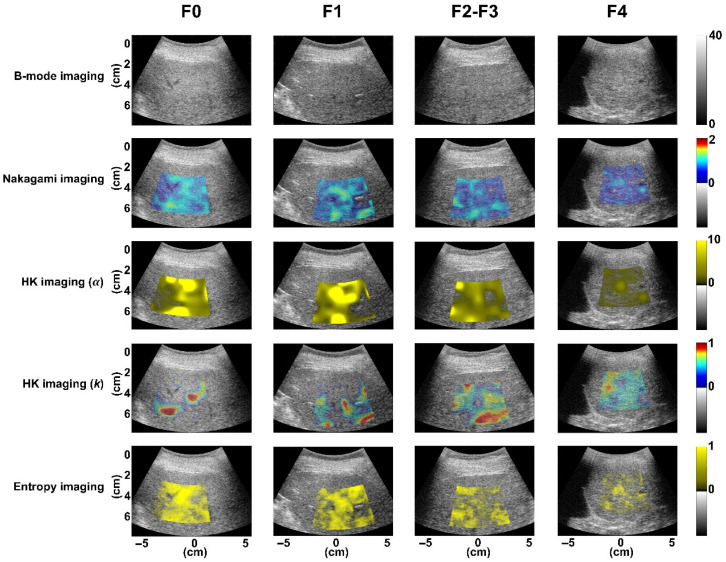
Ultrasound B-mode and QUS envelope statistics imaging (*m*, *α*, *k*, and *H* parametric images) from various stages of liver fibrosis. QUS envelope statistics imaging showed a tendency for decreased brightness as the stage of liver fibrosis advanced.
